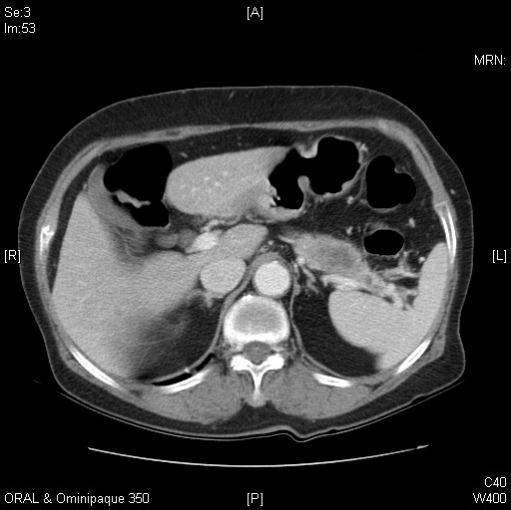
Abdominal computed tomography reveals an ill-defined hypodense mass approximately 3.0 × 1.6 cm in diameter in the pancreatic tail.
